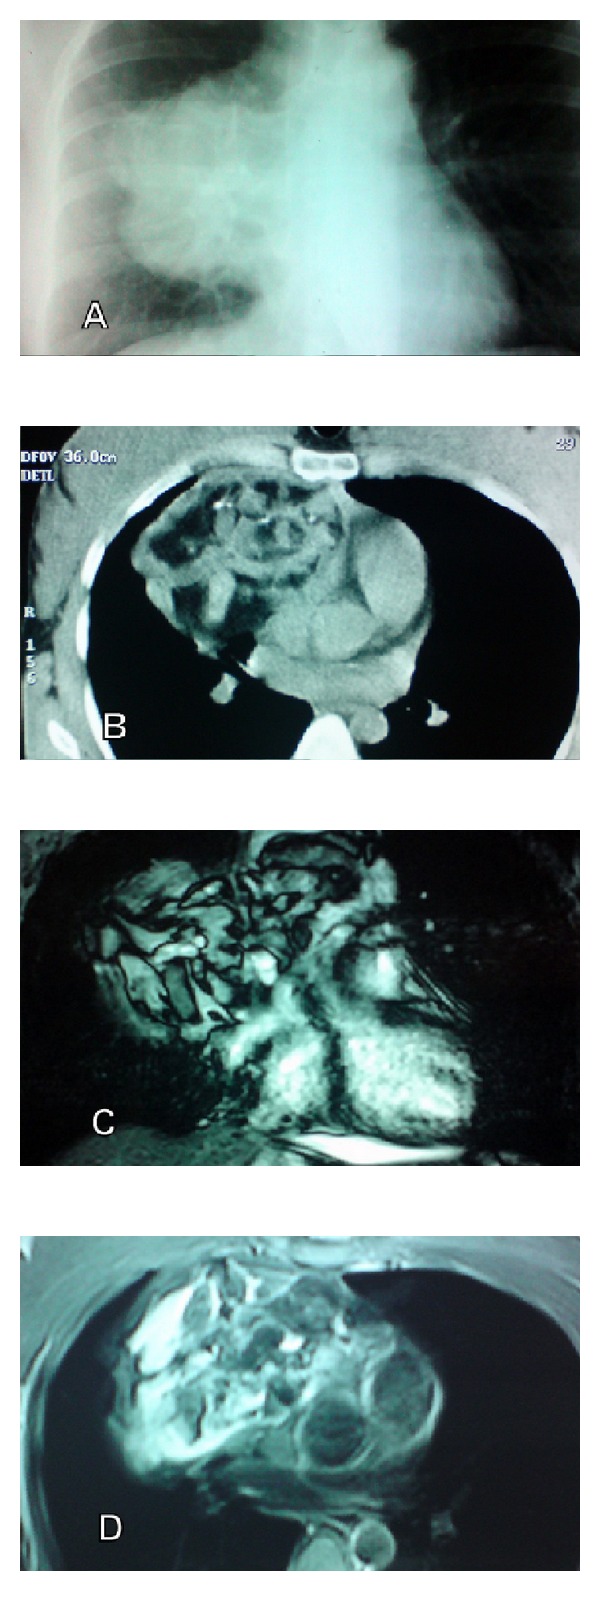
(A) Mediastinal lesion on chest radiography.(B) A mass with heterogenousdensity andcalcificationsin anterior mediastinum onthorax CT.(C) and (D), heart and main vascular structures are displaced by the mass on thoracic MRI.
